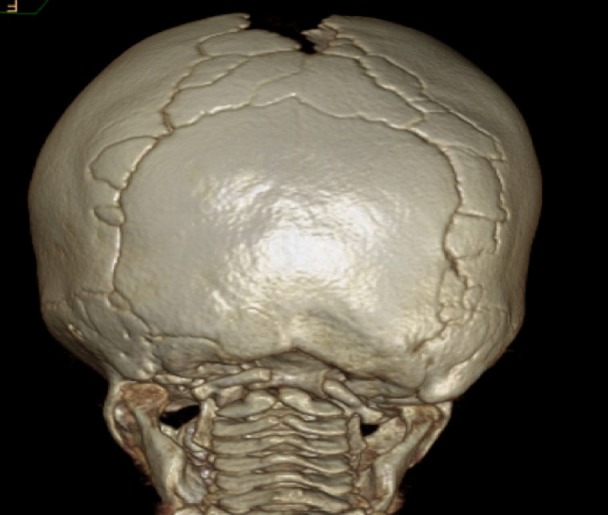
3D head computed tomography (CT) of patient with craniosynostosis Extreme example of sutural bones in the lambdoidal, sagittal, and asterion as seen in a patient with craniosynostosis (bilateral coronal synostosis) on 3D head CT.
